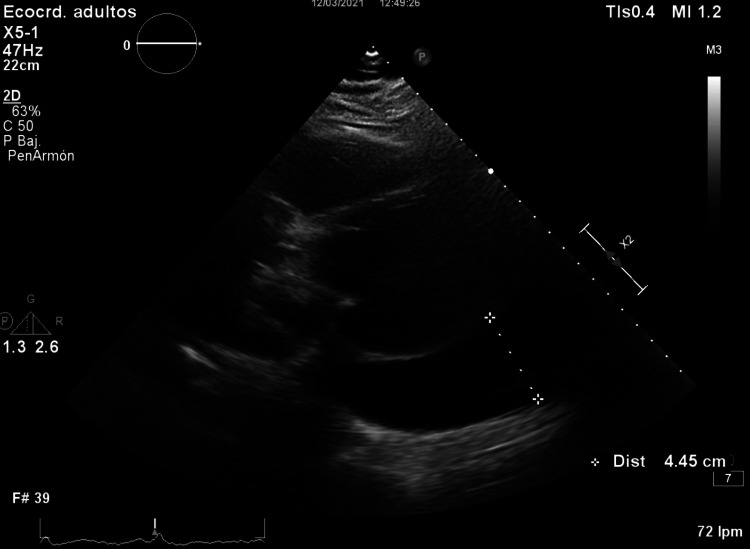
Ultrasound image showing severe pleuropericardial effusion.
